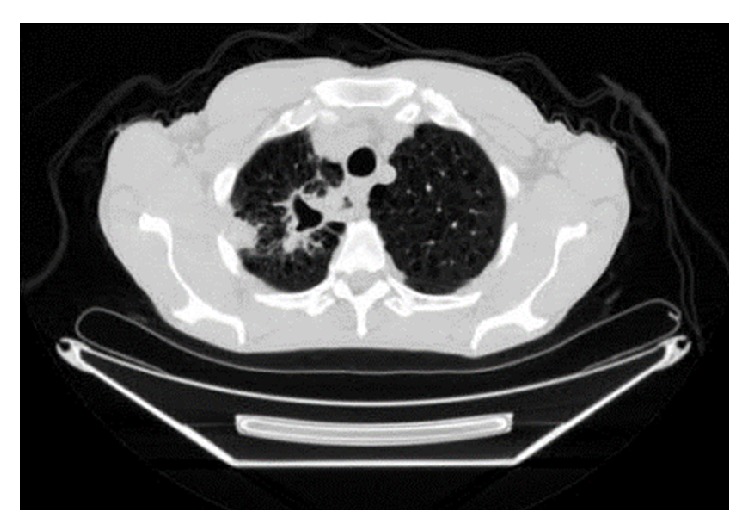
Axial view of CT chest showed a peripheral consolidation (1.5 × 2 × 1 cm) and an apical cavitary lesion (2.5 × 3 × 3.5 cm) in the right upper lobe.
